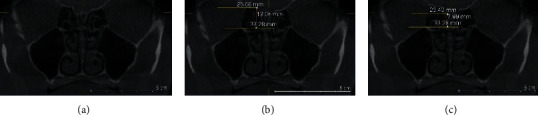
(a) Coronal plan of CBCT demonstrating type 2 TMS classification on the right side. (b) OFL-ER measurement and (c) OFL-CP measurement coronal plan of CBCT.
